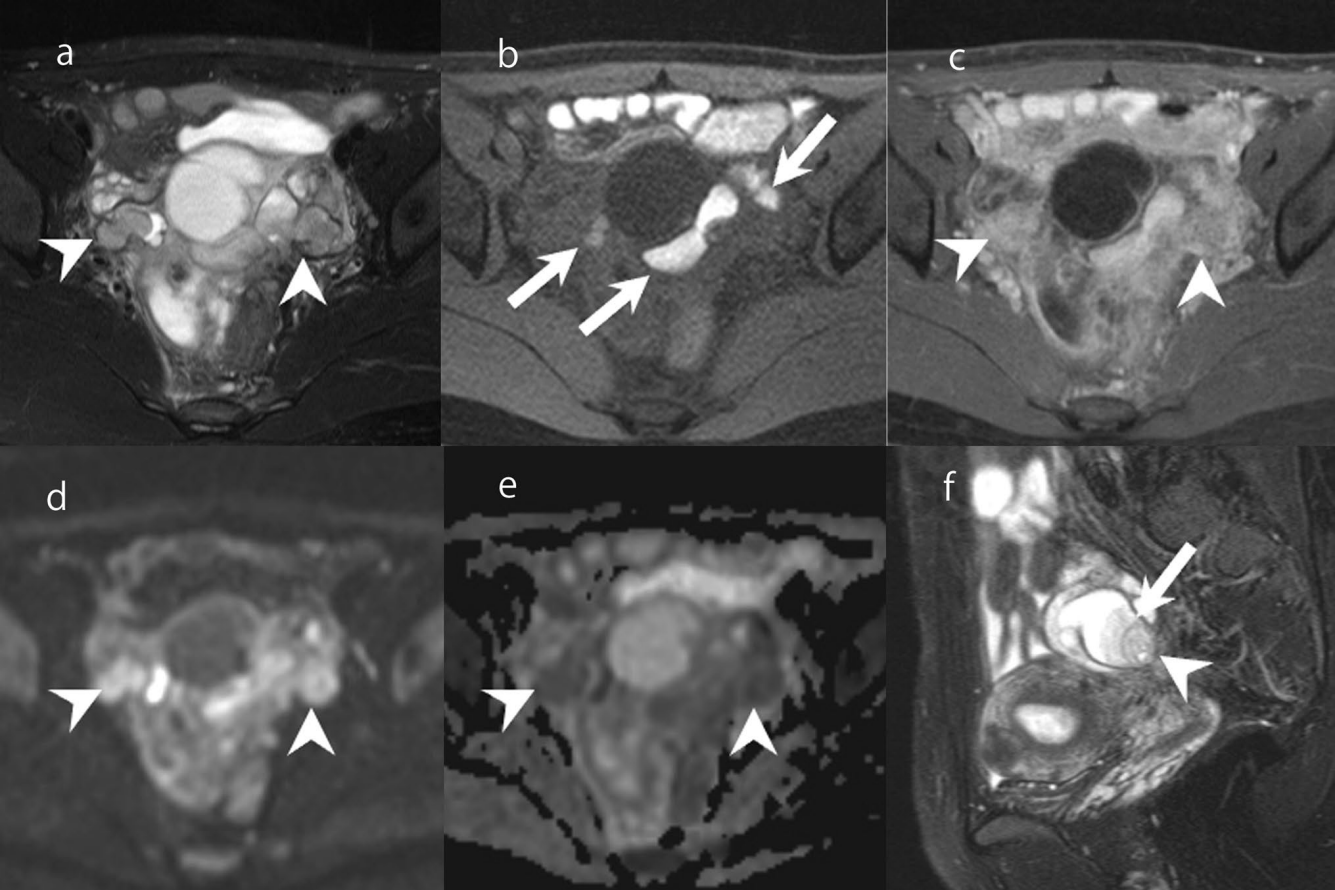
Polypoid endometriosis in a woman in her 30s. a A lesion consisting of cystic and solid components is visible in the pelvis. The solid components are moderately hyperintense on FS-T2WI (arrowhead). b Some of the cystic components are markedly hyperintense on fat-suppressed FS-T1WI (arrow). c Solid components have moderate enhancement on Gd-enhanced FS-T1WI (arrowhead) and are hyperintense on DWI (d: arrowhead). e On ADC maps, the solid components are iso-intense compared with skeletal muscle (arrowhead). f Sagittal FS-T2WI shows the solid component as iso-intense compared with endometrium (arrowhead), and there is a hypointense rim on the surface of the solid component (arrow)
